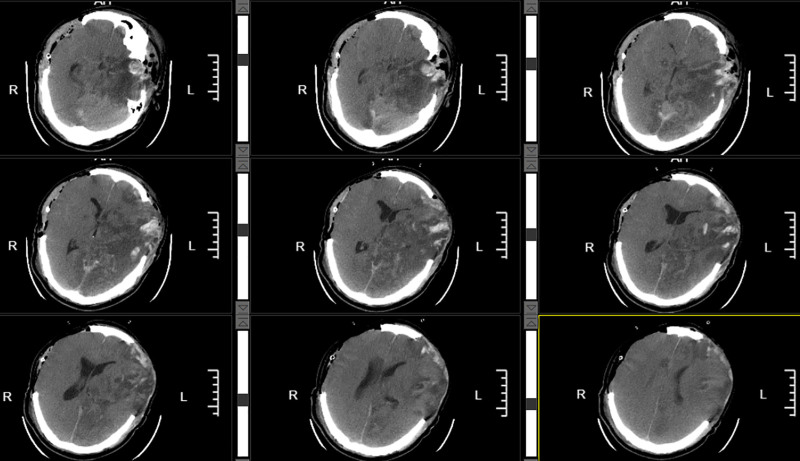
CT imaging demonstrated essentially preserved midline structures, faintly discernible ambient cisterns, and evidence of alleviated intracranial hypertension. CT = computed tomography.
